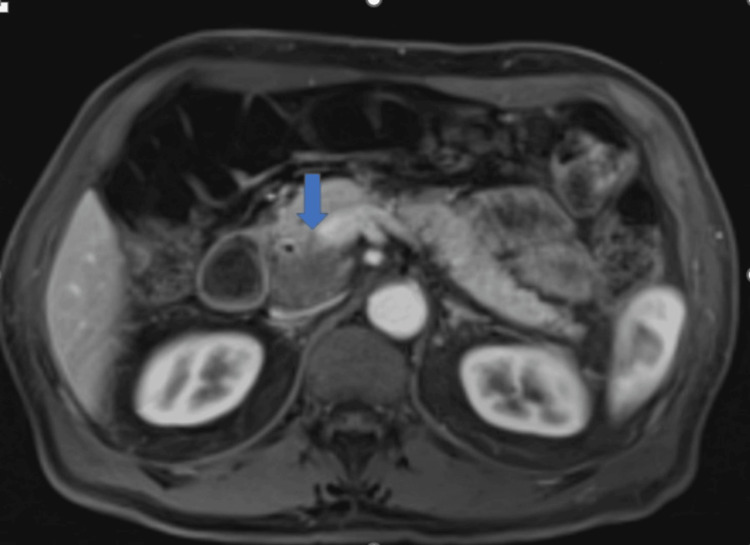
Coronal T2-weighted MRI showing a hypo-enhancing mass in the pancreatic head measuring 51 mm exhibiting restricted diffusion (blue arrow).
